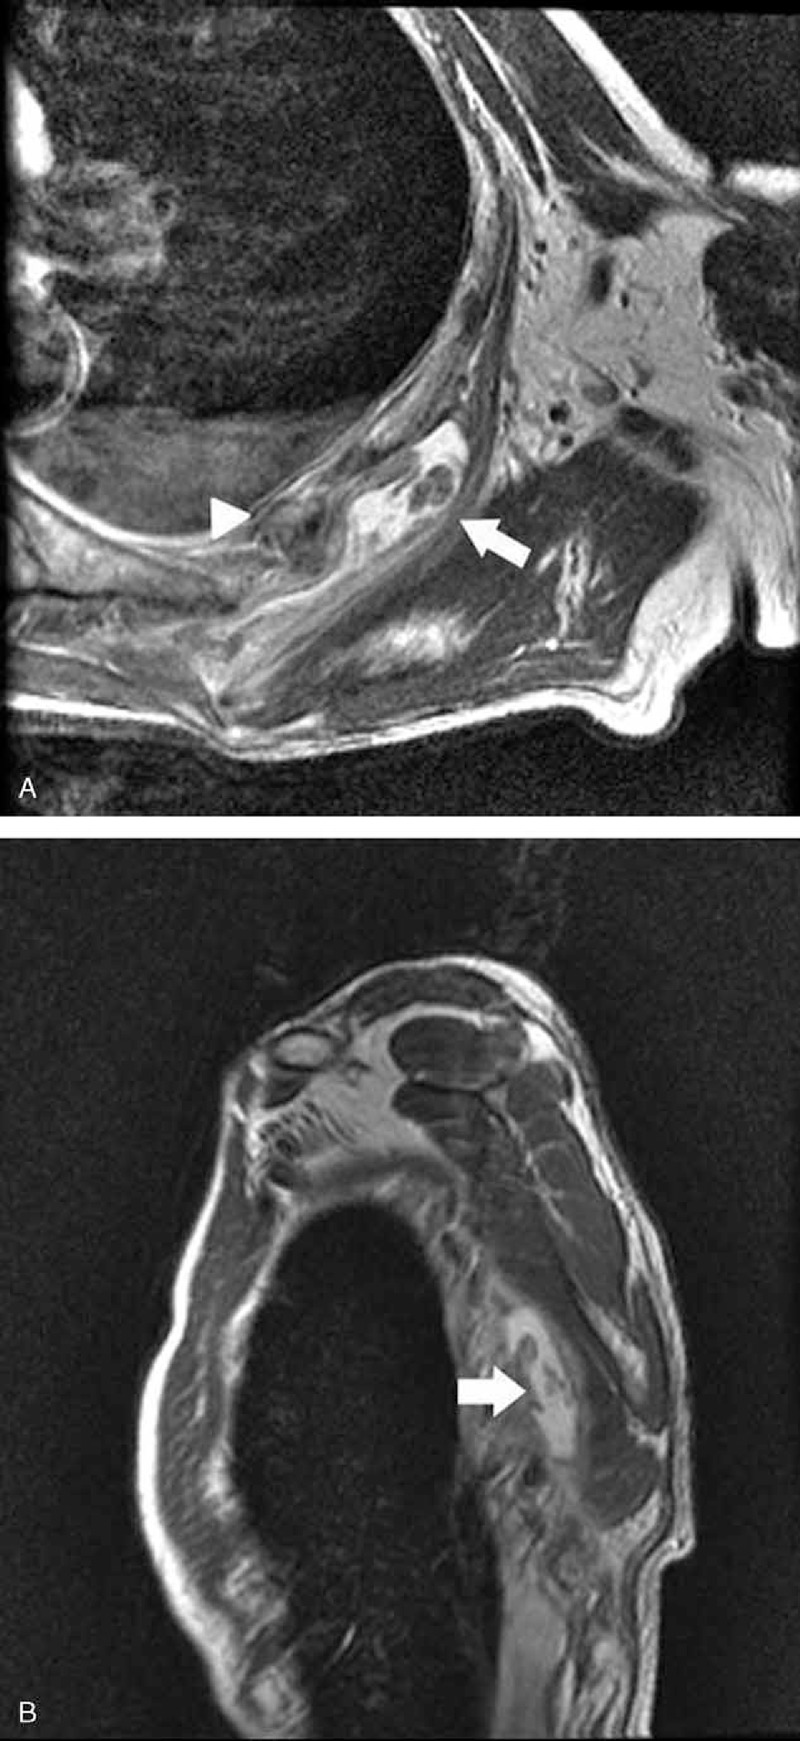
High signal intensity in enlarged scapulothoracic bursa (arrow) and fractured rib (arrowhead) in (A) axial and (B) sagittal view of T2-weighted magnetic resonance images.
